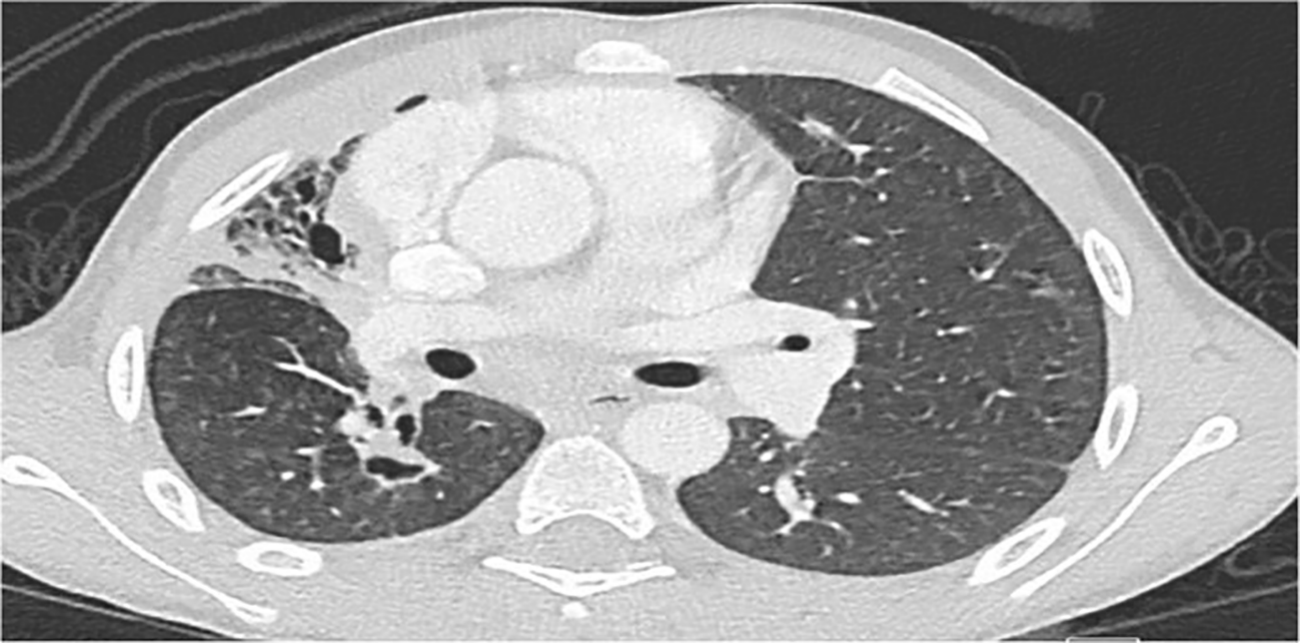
Chest CT in P8 shows marked volume loss and honeycomb pattern in the parenchyma involving almost the entire right upper lobe, associated with pleural thickening. Linear densities in the right lower lobe and left upper lobe suggestive of fibrotic changes. Small cavitating lesion in the apex of the right lower lobe is noted.
